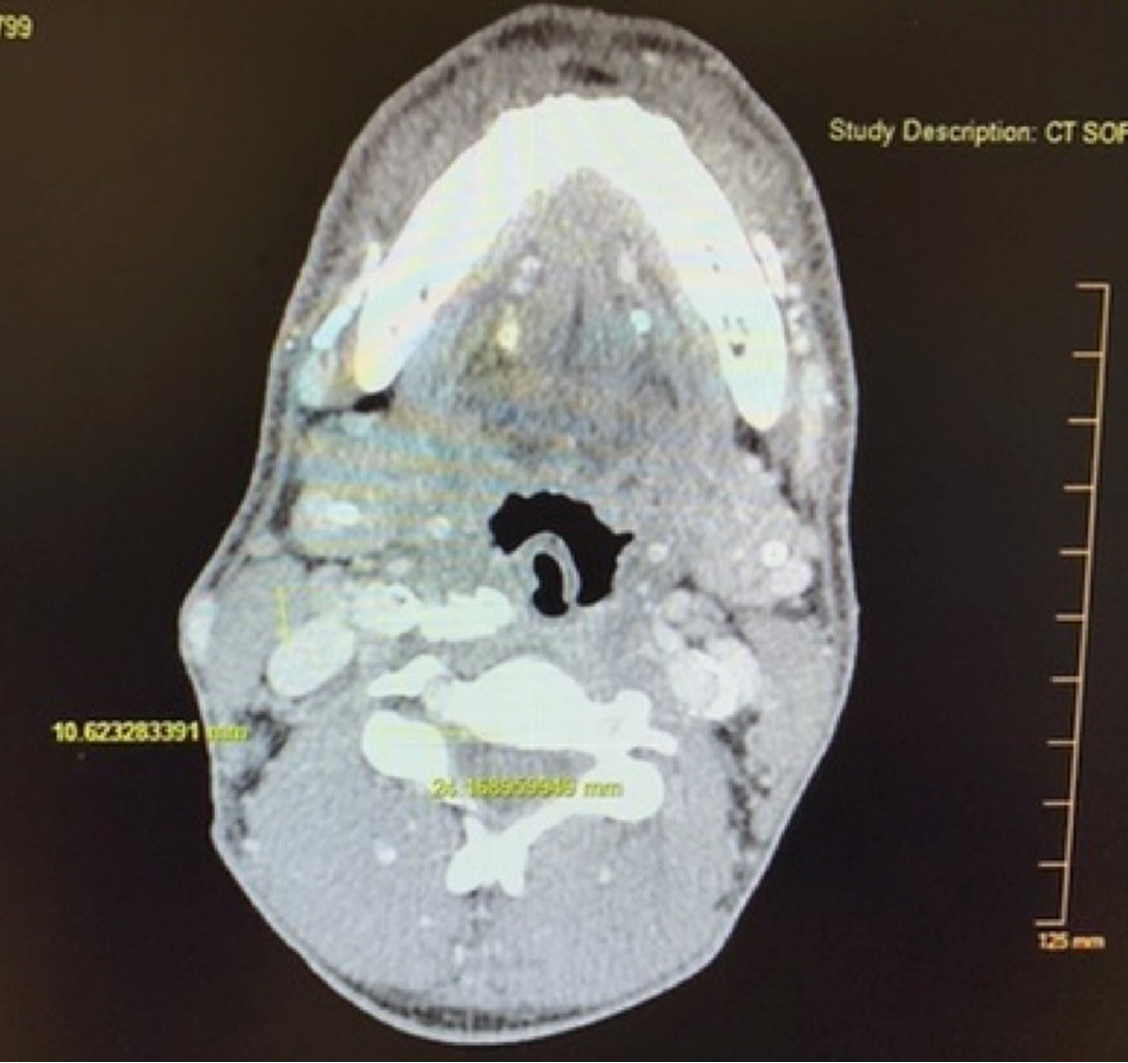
Axial view, CT soft tissue neck. A large calcified lesion in the retropharyngeal area on the right side directly abutting the internal carotid artery
